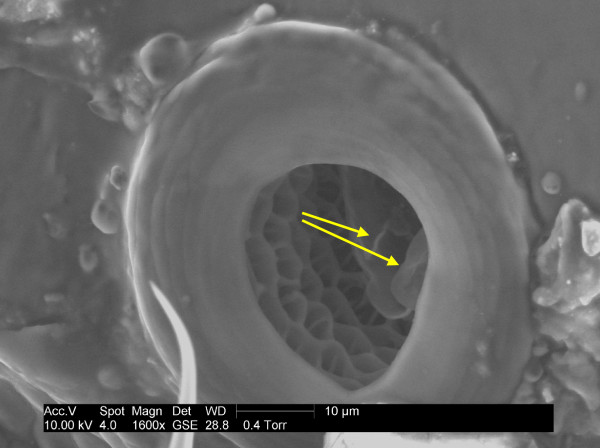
Untreated thoracic spiracle showing secreted material. SEM of an untreated spiracle to show a deposit within the atrium of secreted material of louse origin (arrowed). X-ray analysis showed this deposit was free from silicon.
